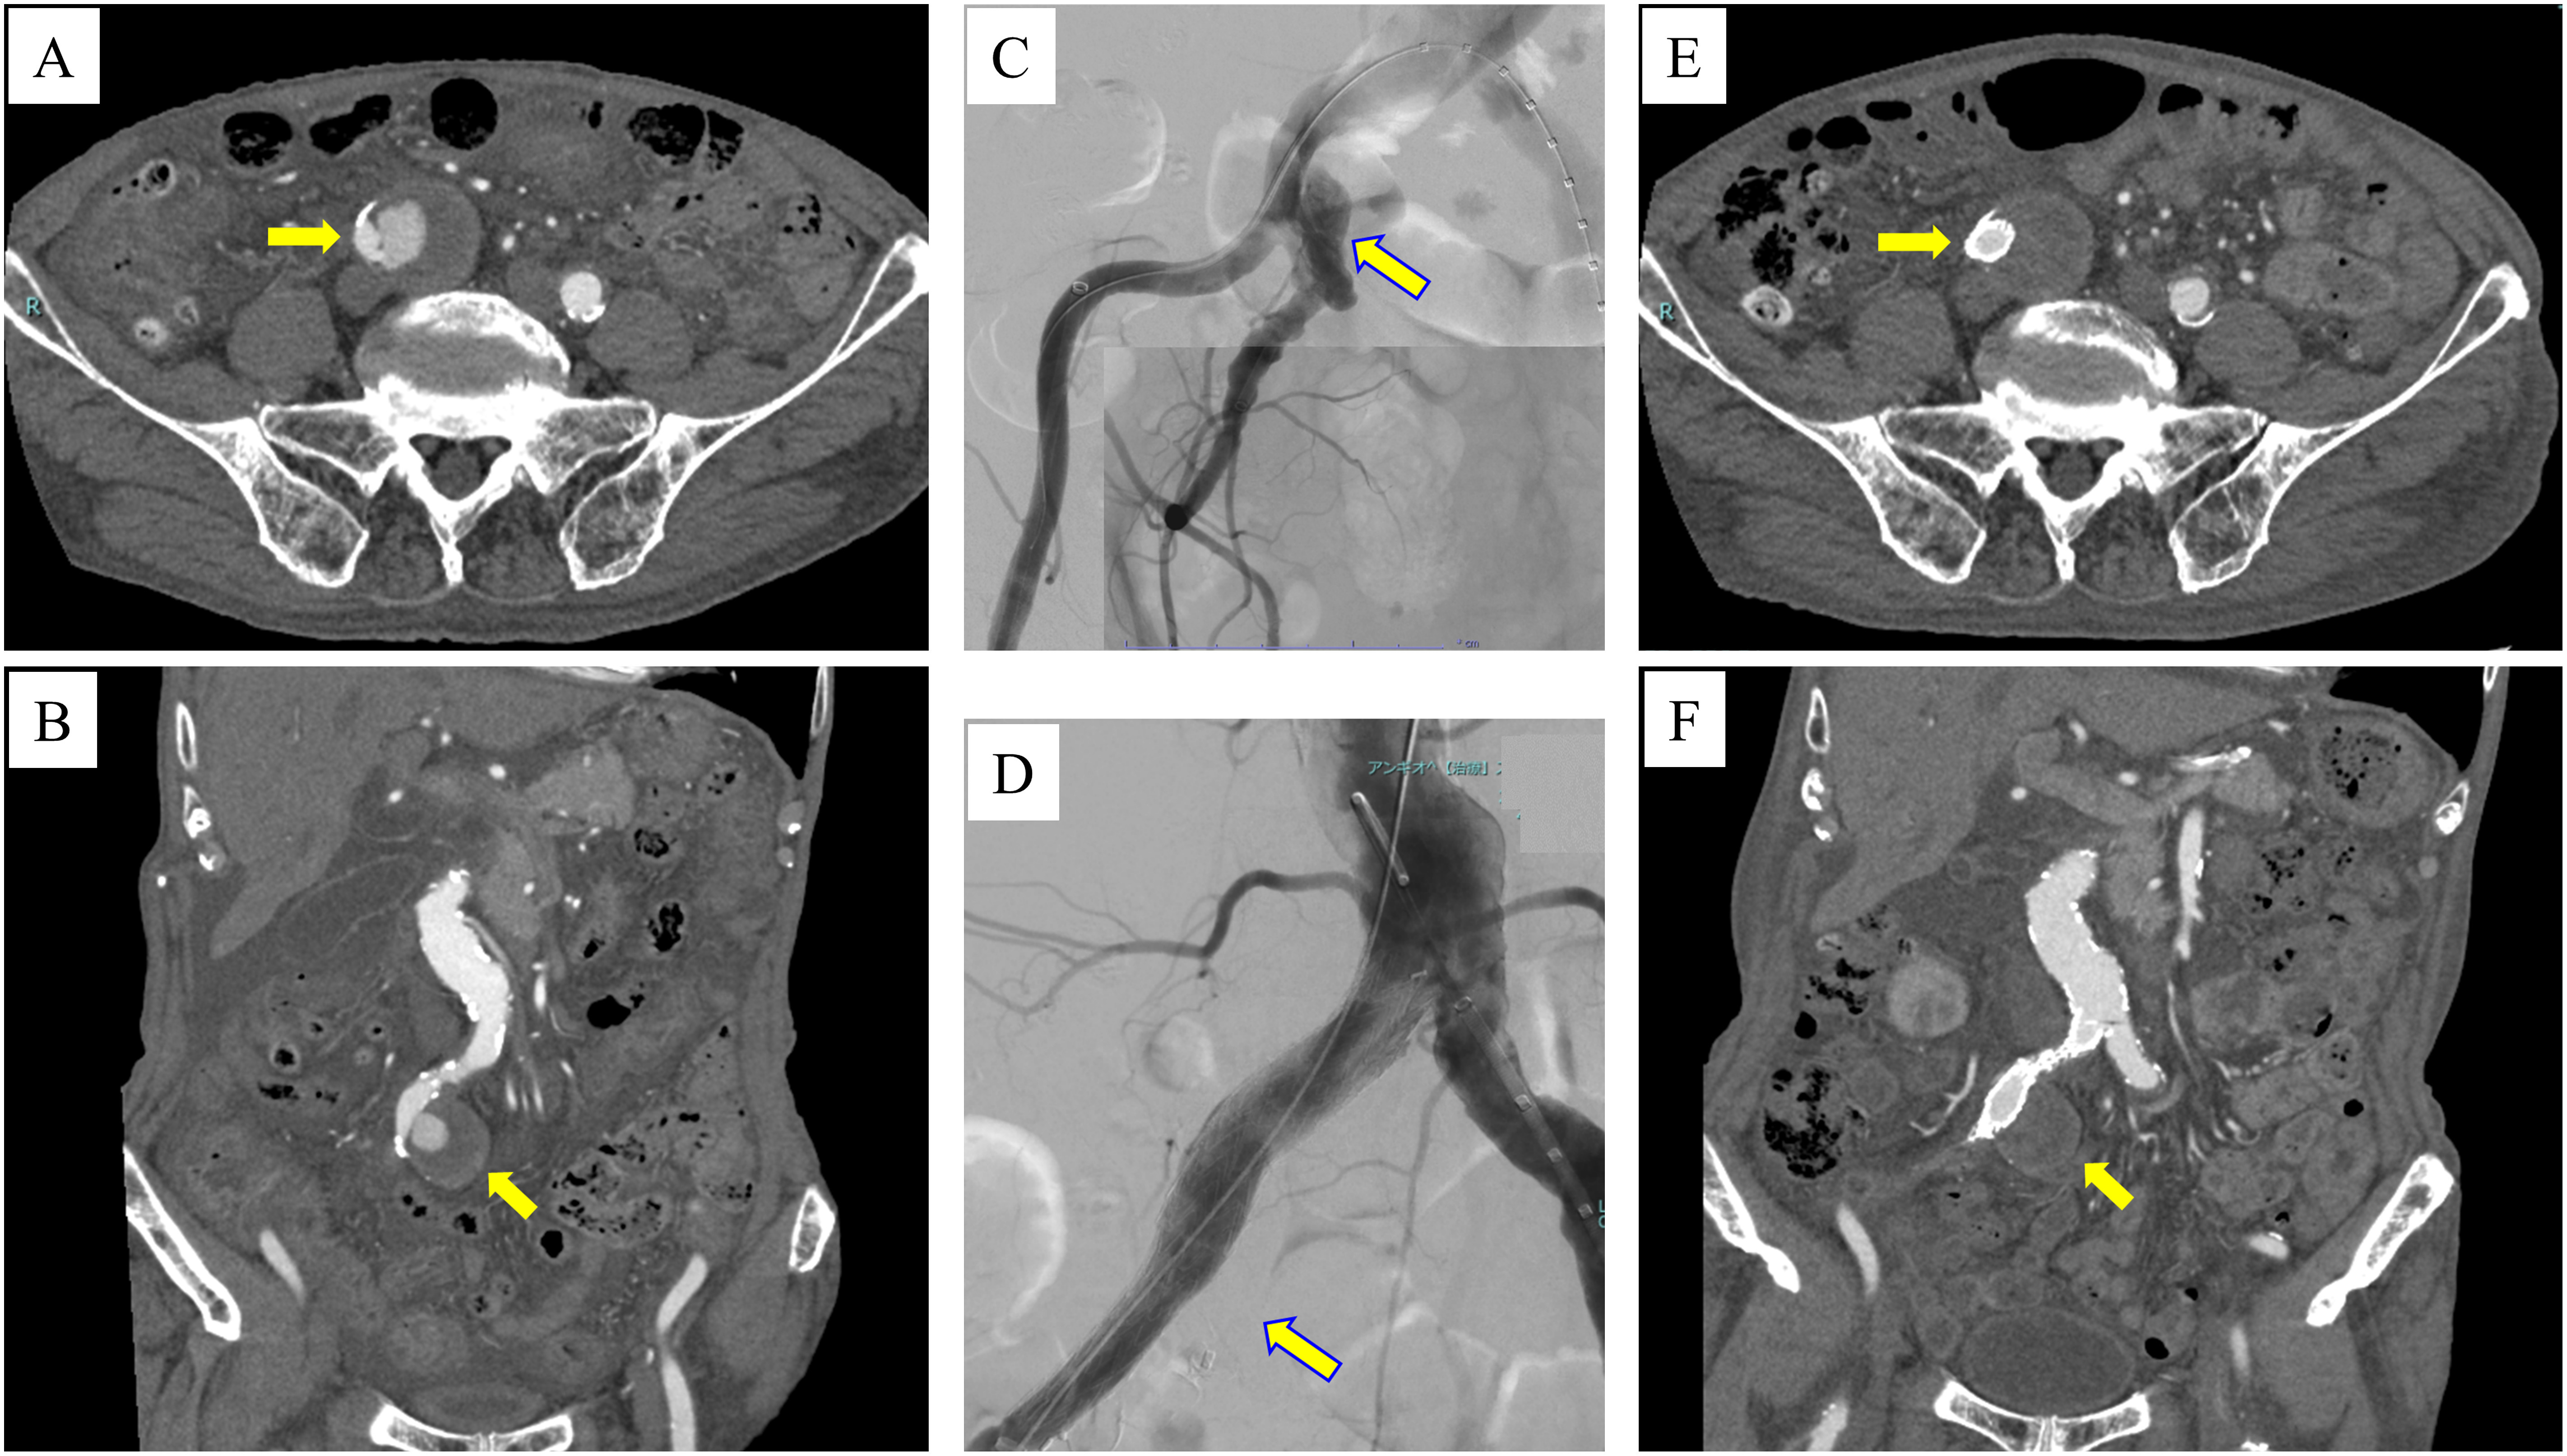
Fig. 1 (A), (B) Computed tomography (CT) showing a 36 mm right internal iliac artery (IIA) aneurysm. (C) Preoperative angiography showed IIA aneurysm. (D) Angiography at initial treatment after IIA embolization and stent graft placement showed no endoleak. (E), (F) The right IIA aneurysm was treated by IIA embolization and stent graft placement. CT 5 days after endovascular treatment showed no obvious endoleak.
